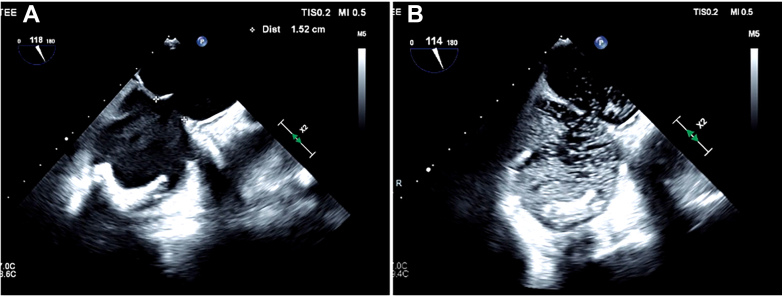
Intraprocedural TEE Assessment of the Septal Defect (A) ASD measuring 1.52 mm on TEE. (B) Agitated saline study after TTViV showed a bidirectional shunt across the ASD. ASD = atrial septal defect; TEE = transesophageal echocardiography; TTViV = transcatheter tricuspid valve-in-valve.
